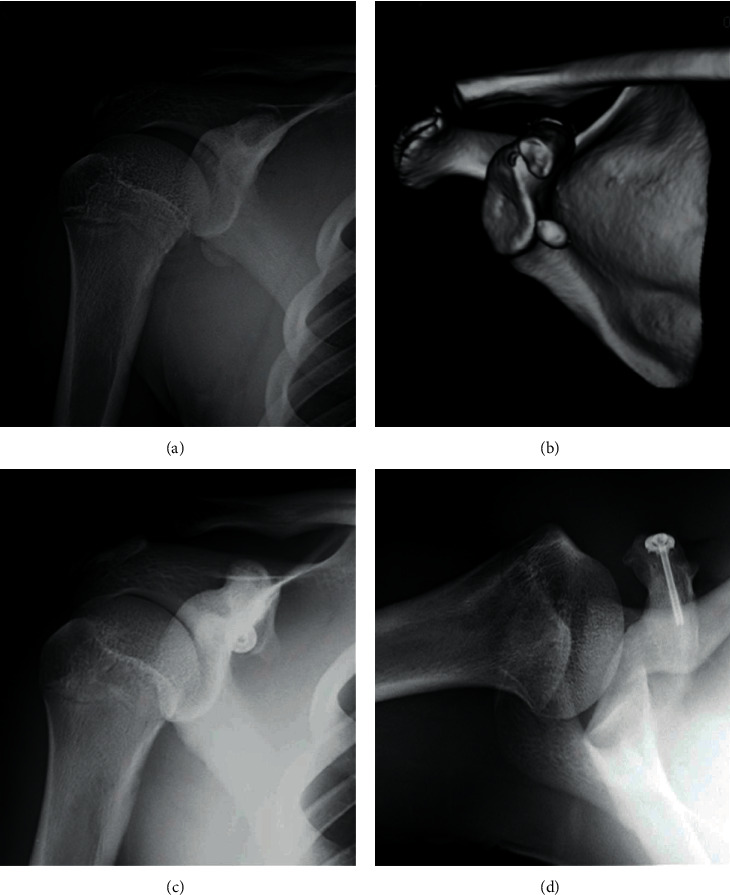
Representative anteroposterior radiograph: three-dimensional computed tomography reconstruction (a), Type III injury (b), anteroposterior radiograph two years postoperatively (c), and axillary radiograph two years postoperatively (d). This image was used with permission from Kurume University Medical Center and Dr. K. Nakama [21].
